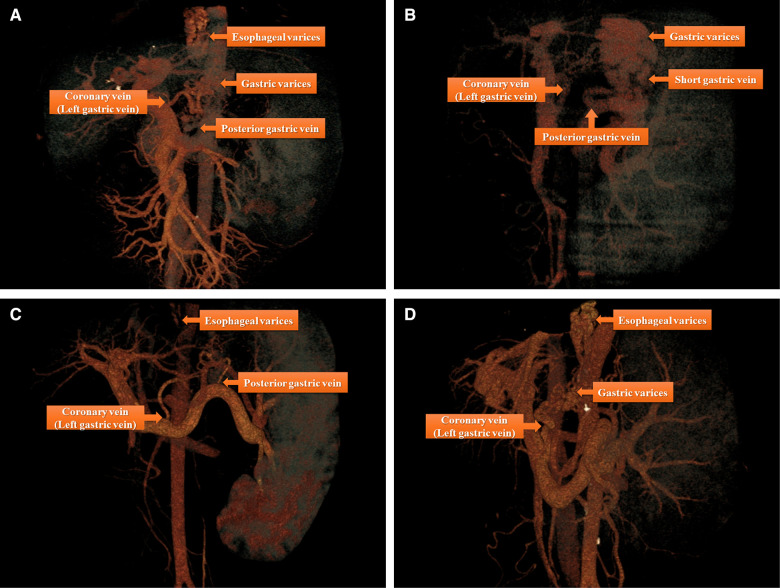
There are four major types of anatomical variations of portal vein system by three-dimensional images reconstruction based on the data derived from 64-slice spiral CT: (A) Esophageal and gastric varices (the source of bleeding) were found to flow into coronary vein (left gastric vein) and posterior gastric vein; (B) gastric varices (the source of bleeding) were found to flow into posterior gastric vein and short gastric vein; (C) Esophageal varices (the source of bleeding) were found to flow into coronary vein (left gastric vein) and posterior gastric vein; (D) Esophageal and gastric varices (the source of bleeding) were found to flow into coronary vein (left gastric vein).
